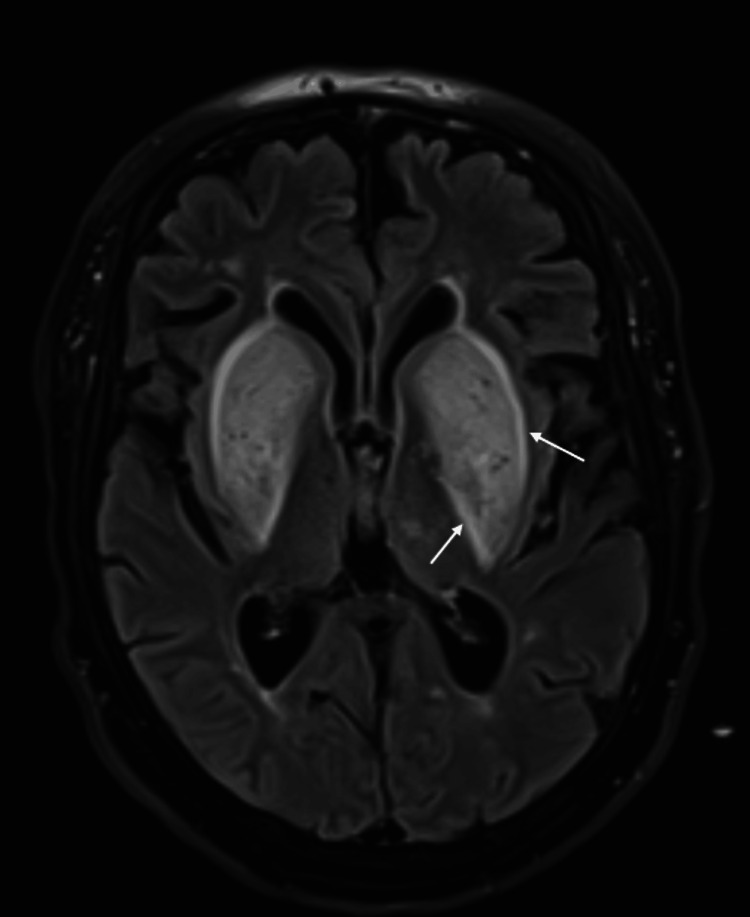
Axial T2-weighted FLAIR imaging of the brain Axial T2-weighted fluid-attenuated inversion recovery (FLAIR) imaging demonstrates bilateral symmetrical swollen lentiform nuclei with a hyperintense rim delineating the lentiform nucleus with a typical fork-like appearance representing the “lentiform fork" sign (arrow).
